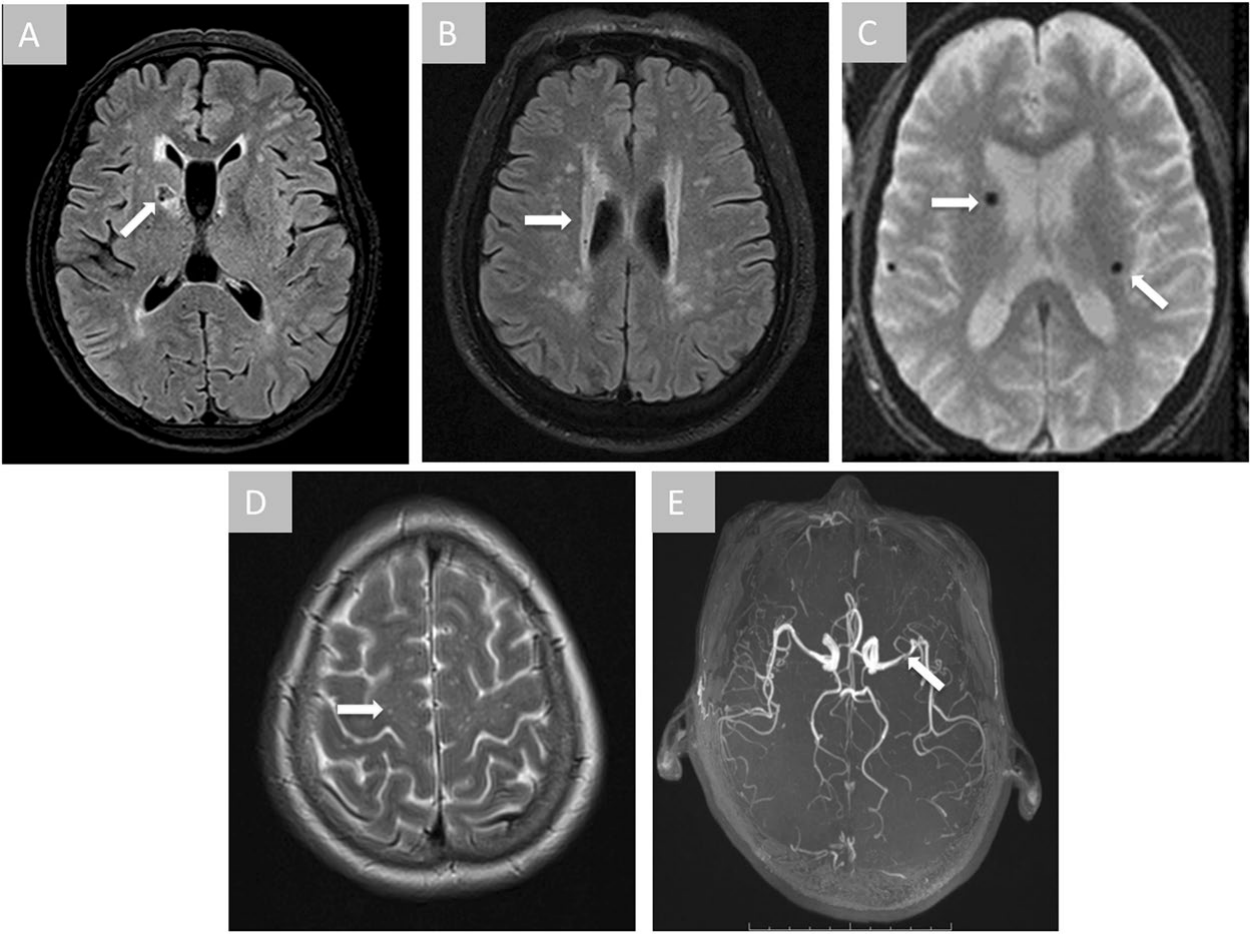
Incidental findings on brain MRI. Arrows indicate the abnormalities in each image. Panel A, lacunes; Panel B, white matter hyperintensity; Panel C, cerebral microbleeds; Panel D, perivascular space; Panel E, intracranial arterial stenosis.
